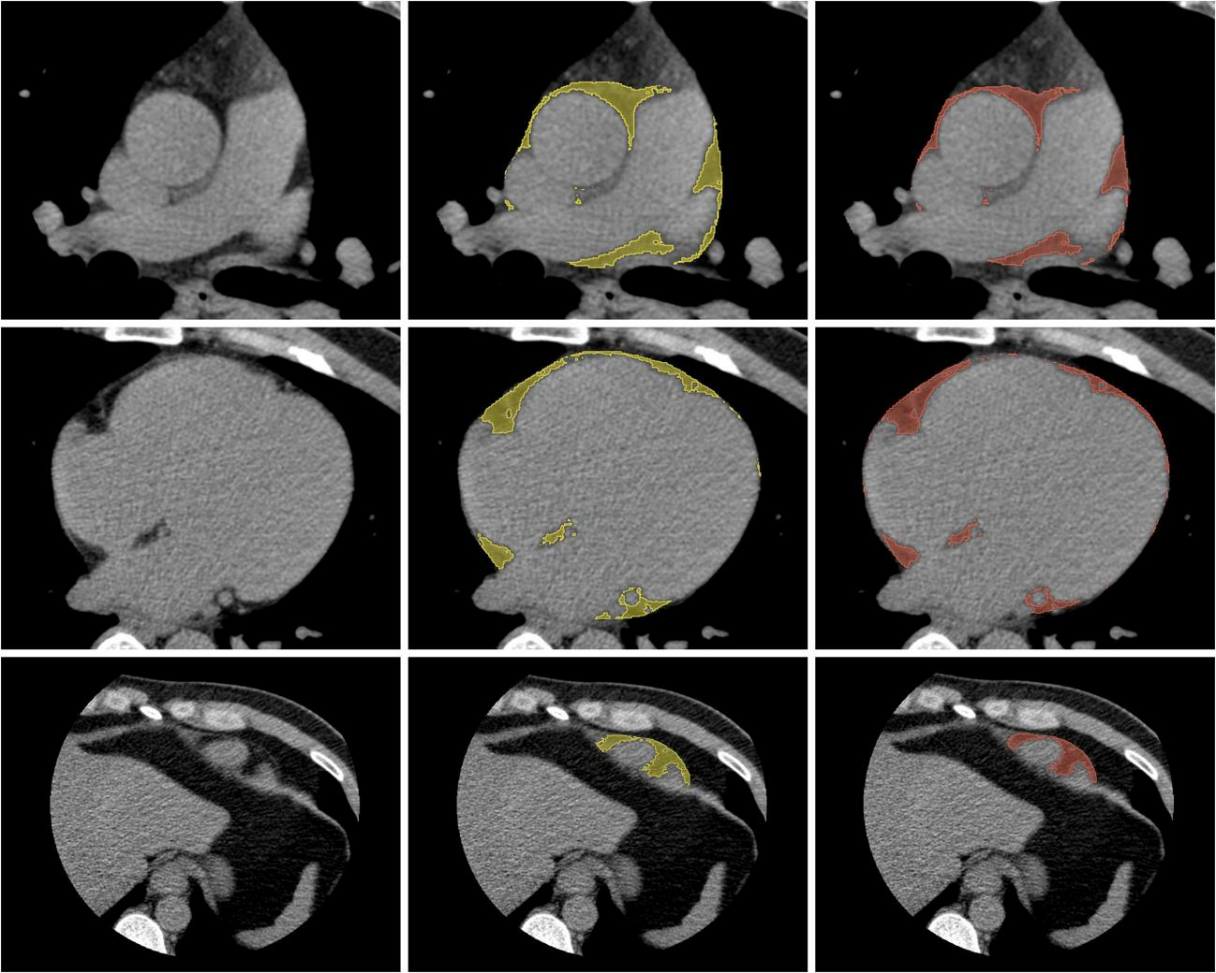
Comparison of human annotations (middle column) and AI predictions (right column) for epicardial adipose tissue at the superior limit of the heart (top row), the mid-ventricular slice (middle row), and the inferior limit of the heart (bottom row). The left column shows the corresponding non-contrast computed tomography images.
